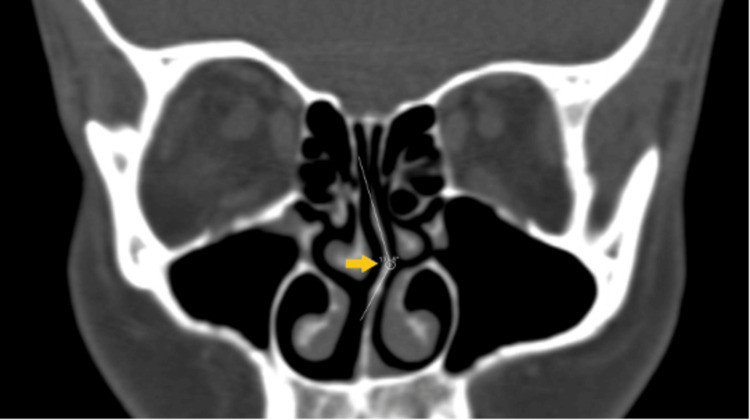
Coronal computed tomography scan showing the measurement of the septal deviation curve angle A relevant anatomical alteration in patients with nasal obstruction was noted. Figure reproduced from Bekin Sarikaya P, Bayar Muluk N. *Relationship between nasal septal deviation angles and turbinates: a computed tomography study*. Cureus. 2023;15(2):e35253. Licensed under CC BY 4.0 [41].
